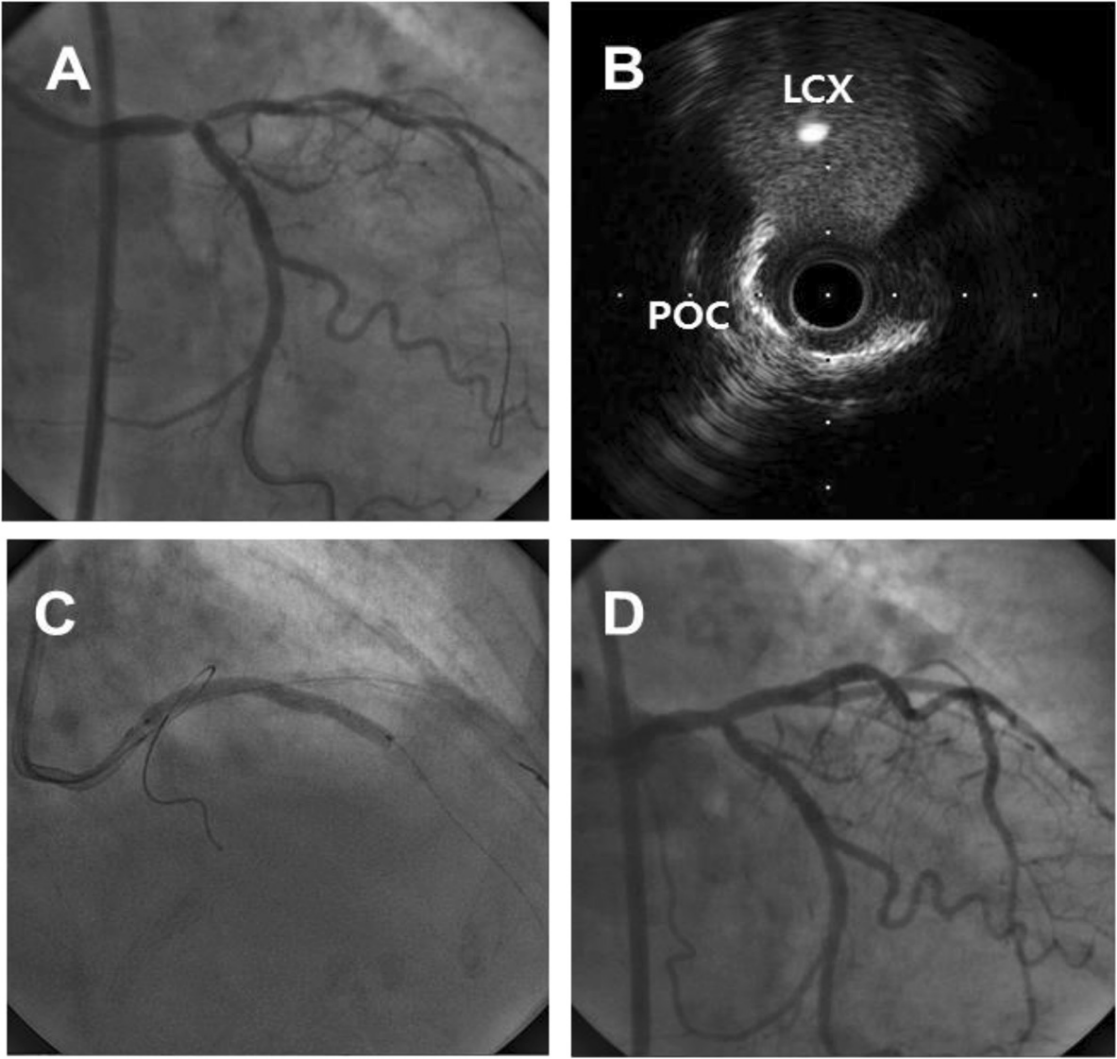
Provisional approach for distal left main stenosis. Coronary angiography showed a “true” LM bifurcation lesion (Medina 1.1.1) (a) while intravascular ultrasound revealed very minimal disease at the ostium of the left circumflex artery (b). Provisional single stenting was performed (c), with the final angiogram showing an acceptable result without side branch compromise (d)
